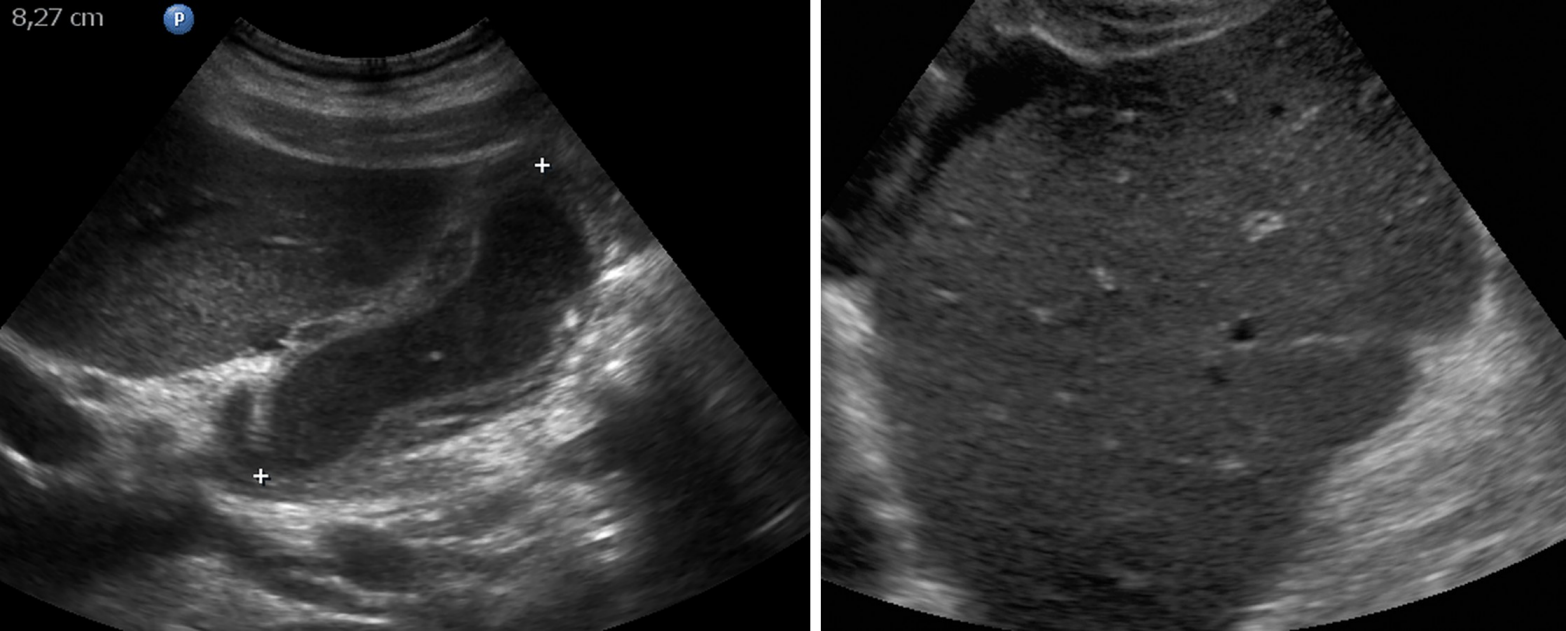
Signs of acute hepatitis. (A) Pronounced thickening of the gallbladder wall, filled with sludge. This represents a typical sonographic finding in our study. (B) Indicated starry sky appearance of the liver with decreased echogenicity of liver parenchyma and pronounced bile ducts.
